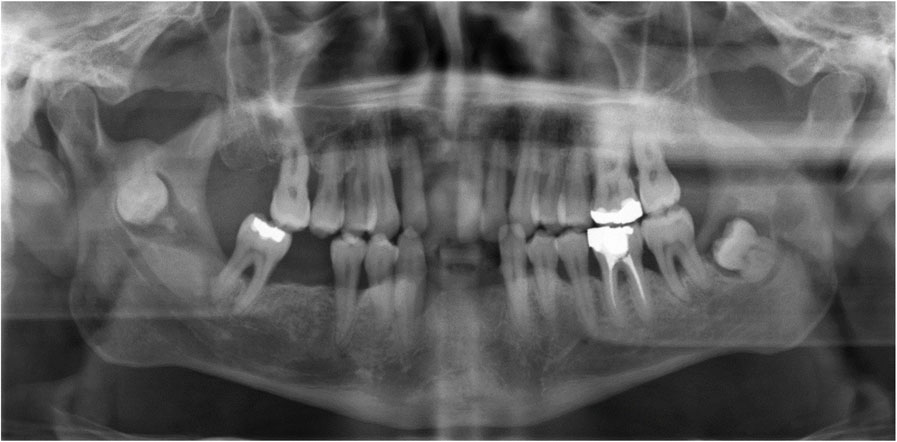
Panoramic radiograph showing the ectopic third right molar
